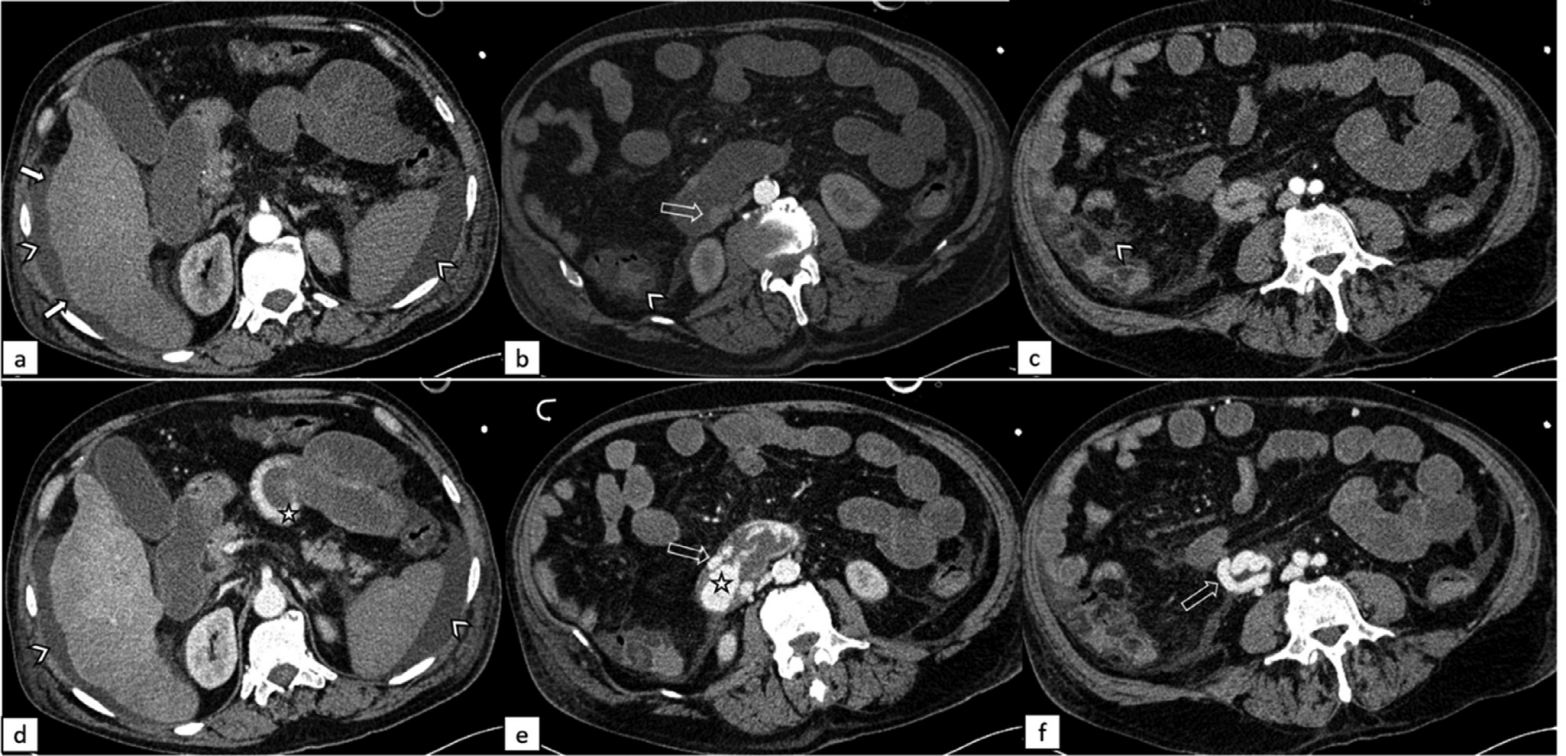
Contrast enhanced computed tomography of the abdomen. Same three slices in the arterial phase (above) and portal phase (below), showing signs of cirrhosis (arrowheads: ascites; white arrows: liver surface undulations), venous varices (empty arrows) in the third section of the duodenum (b, e, f) and intraluminal active bleeding (star).
